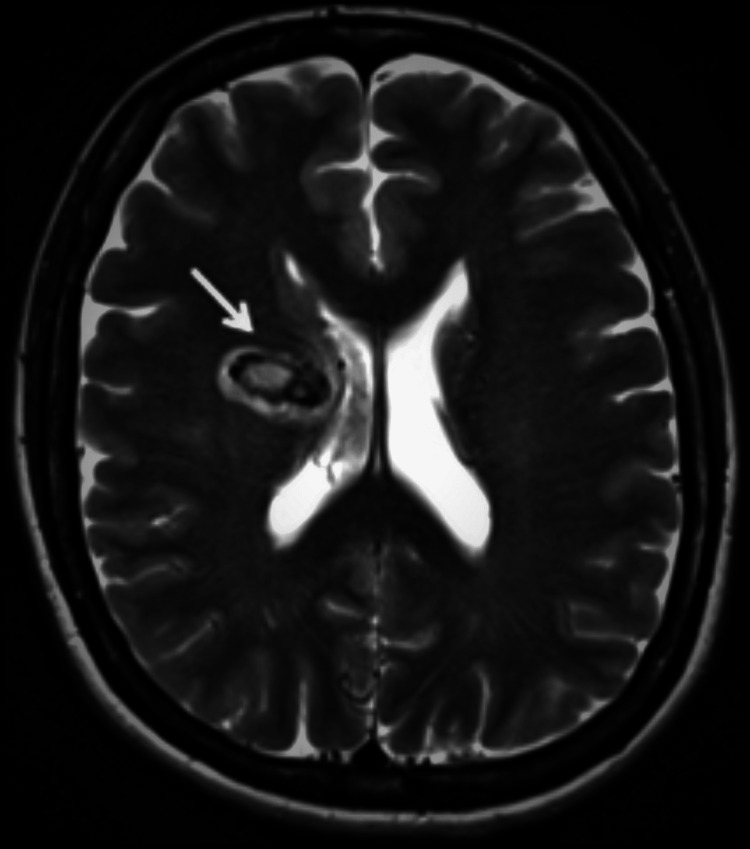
Acute right frontal capsular parenchymal hemorrhage on T2-weighted MRI (arrow) MRI, magnetic resonance imaging
